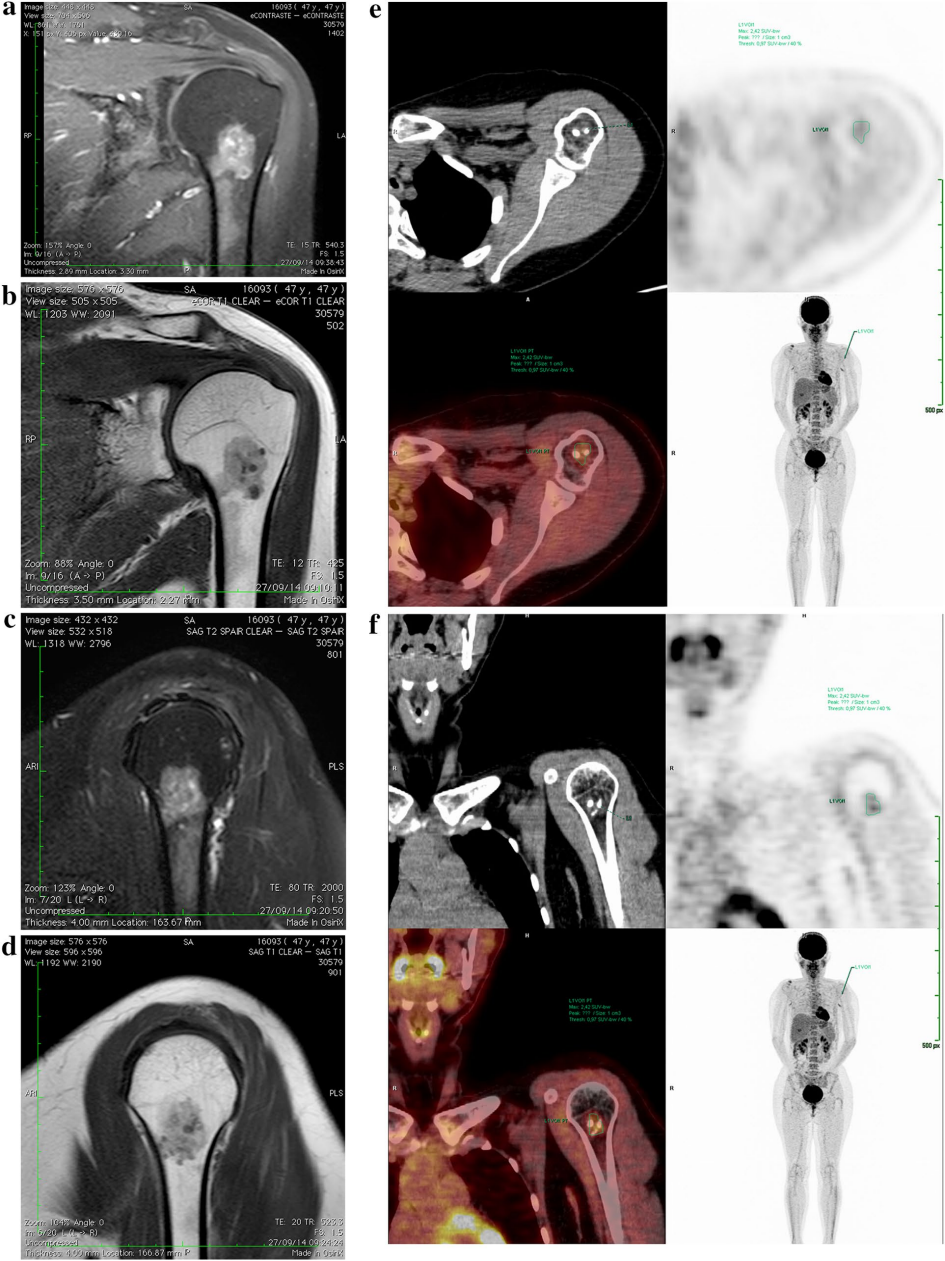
Example of an MRI image of the shoulder, where it is not possible to confirm whether the lesion is a chondroma or a chondrosarcoma. In a axial T2 MRI image of the proximal humerus, and b T1 image. In c and d, the bone lesion can be visualized in T2 and T1 images. In e, axial PET and in f coronal images show the proximal region of the left humerus. Note the PET–CT presenting the volumetric region of interest (VOI) with an SUVmax = 2.0
